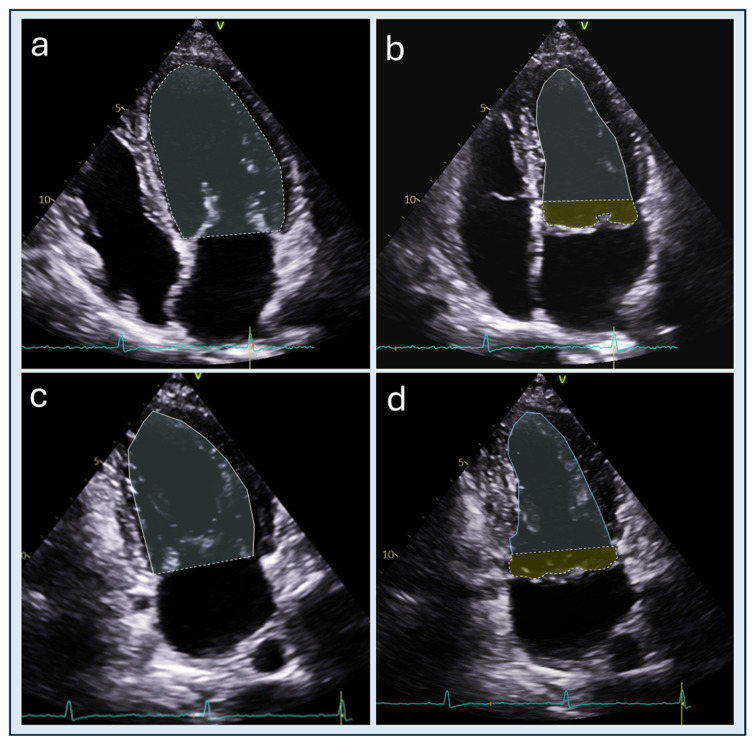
Apical four-chamber and two-chamber views used for biplane volumetric assessment of left ventricular volumes and ejection fraction according to the modified Simpson’s rule. Four-chamber view at end-diastole (a) and at end-systole (b), two-chamber view at end-diastole (c), and at end-systole (d). Endocardial contours are traced excluding the region located above the mitral annular plane. In the presence of mitral valve prolapse, the blood compartment interposed between the mitral annular plane and the ventricular surface of the prolapsing leaflets (doming volume; highlighted in green) is generally excluded from end-systolic contouring, which may lead to underestimation of LV end-systolic volume and consequent overestimation of ejection fraction.
